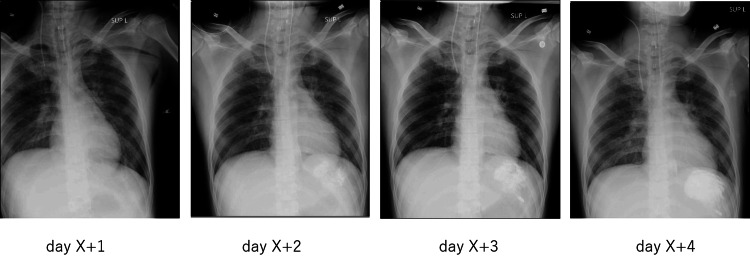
Changes in chest X-ray findings over time after admission The area of high radiopacity on radiography enlarged after the initiation of sodium zirconium cyclosilicate.
